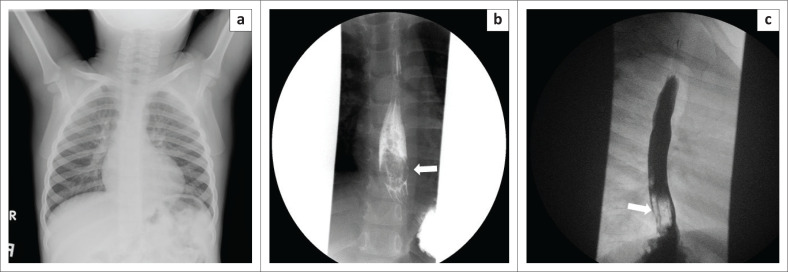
Images of a 3-year-old child who ingested a toy part (toy car tyre). The chest radiograph (a) was unremarkable. Upper oesophagogram, frontal (b) and oblique (c) views, showed a ring-shaped filling defect in the lower oesophagus, immediately proximal to the gastro-oesophageal junction with contrast traversing into the stomach without any delay.
